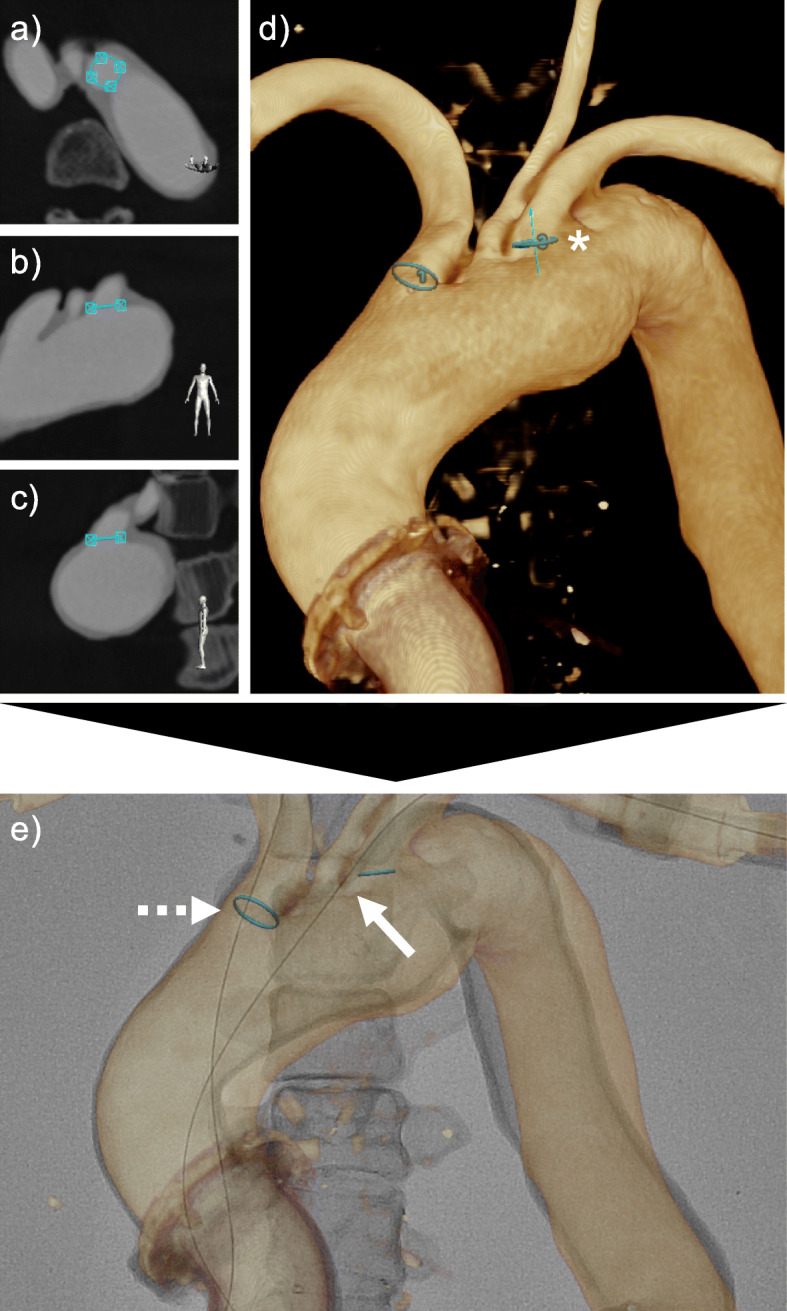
Exemplary placement of circular markers at vessel orifices and accuracy assessment via cannulation of those orifices with a guidewire. A circular marker was carefully adjusted to the anatomy of the left subclavian artery’s orifice using multiplanar reconstructions of the cross-sectional imaging data, in this case, a CTA (a–c). Each plane’s orientation is demonstrated by the human model in the bottom right corner of each image. d Correct placement of the marker (*) was verified in the vessel’s virtual 3D model, which was reconstructed from the original CTA data. For demonstration purposes, another marker was placed in the brachiocephalic trunk. e Subsequently, image fusion was performed, and each orifice was cannulated with a soft guidewire. If the wire went through the circular marker (brachiocephalic trunk; dashed arrow), the marker position was marked as accurate, and if the wire missed the marker (left subclavian artery; straight arrow), the position was dismissed as inaccurate
